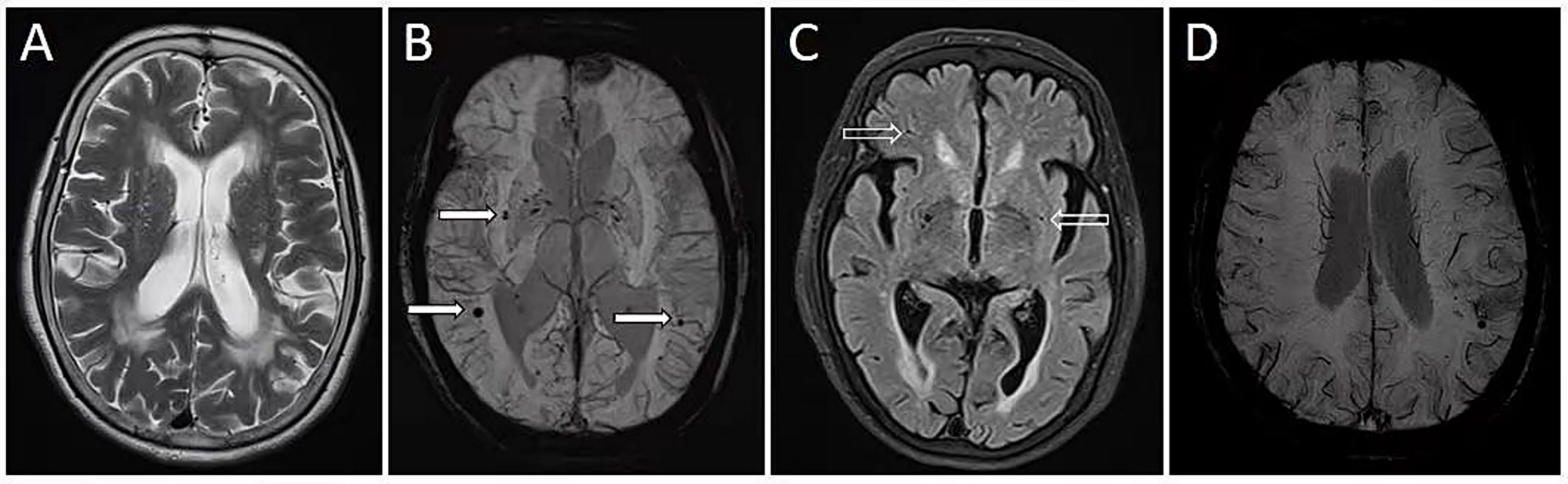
Quantitative neuroimaging markers in a hypertensive patient with cognitive decline. A 71-year-old woman with a history of hypertension was admitted due to cognitive decline. She received a score of 8 on the Montreal Cognitive Assessment. Magnetic resonance imaging (MRI) revealed severe white matter intensities (WMH) (A), cerebral microbleeds (CMB) (B, solid arrows), and lacunar infarctions (LI) (C, hollow arrows). Quantitative analysis using the AccuBrain@ software revealed a WMH volume of 59.7 mL, CMB count of 23, and LI count of 17. MRI susceptibility-weighted imaging also identified discontinuous deep medullary veins (DMV) (D), with a DMV score of 15.
